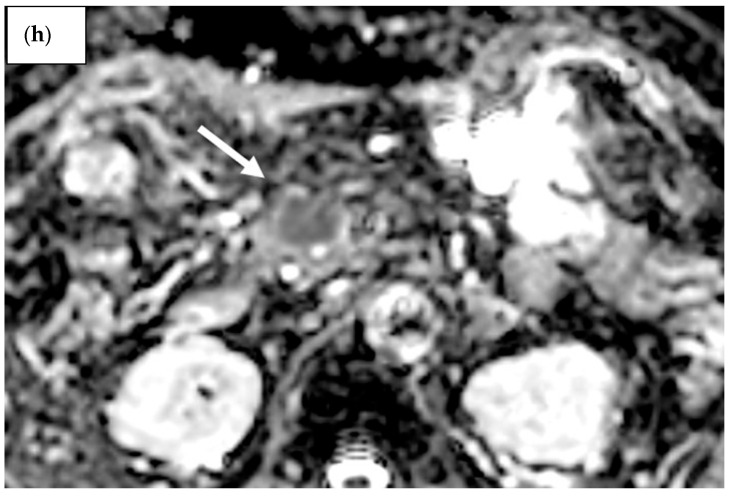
A 59-year-old man with autoimmune pancreatitis (AIP). (a–d) CT images pre-contrast (a) and during the pancreatic phase (b), portal venous phase (c), and equilibrium phase (d). Regions of interest (in black) are manually drawn on the AIP region and abdominal aorta on pre-contrast (a) and equilibrium-phase (d) images. The calculated contrast enhancement was 56.1 HU and the extracellular volume fraction was 55.5%. (e) On pre-contrast, the AIP region located in the head of the pancreas shows low signal intensity compared with normal pancreatic parenchyma (arrow). (f) T2-weighted image with fat suppression. The AIP region located in the head of the pancreas shows slight signal hyperintensity compared with normal pancreatic parenchyma (arrow). (g) Diffusion-weighted imaging (b = 800 s/mm^2^) shows the AIP region with high signal intensity (arrow). (h) The apparent diffusion coefficient is 0.89 × 10^−3^ mm^2^/s (arrow).
